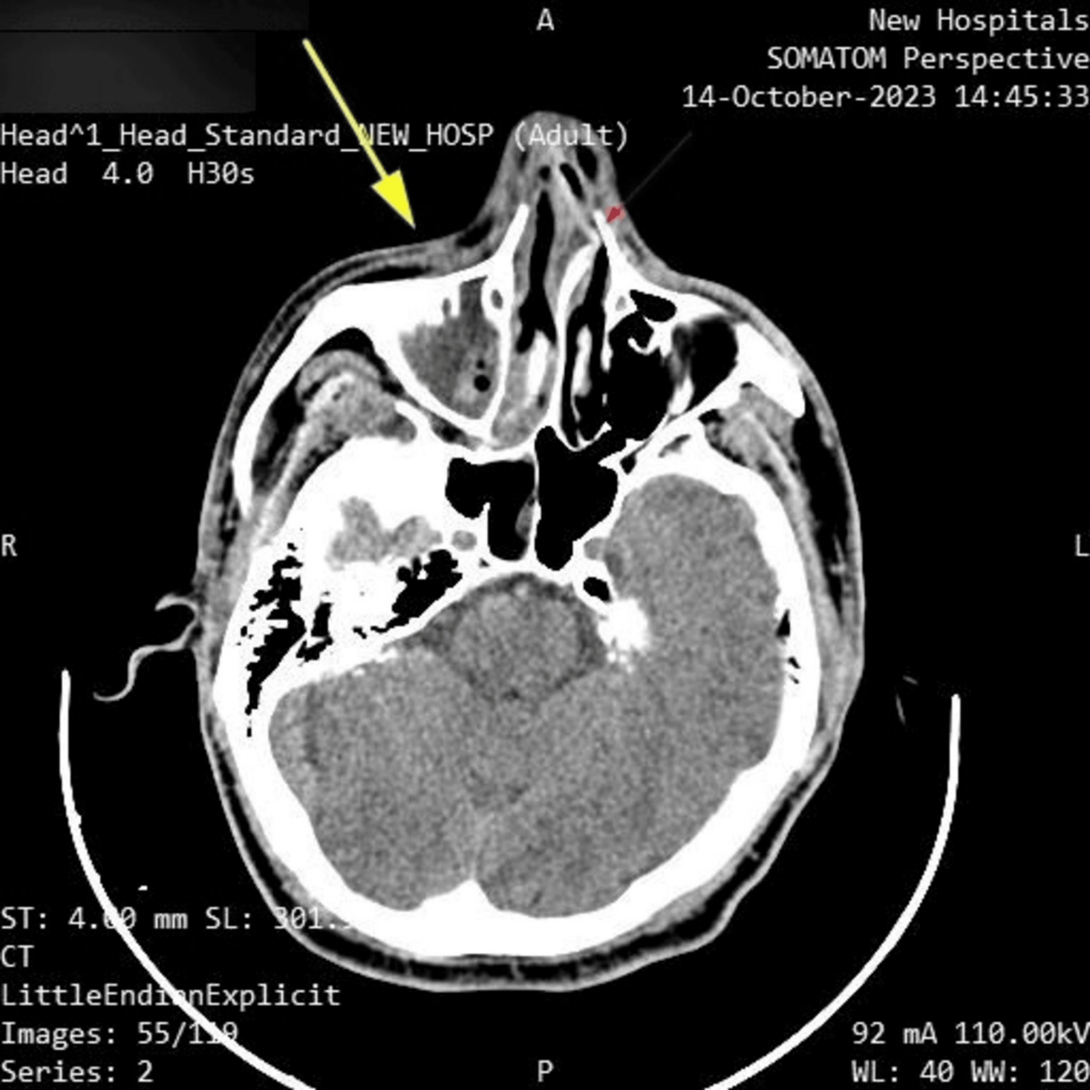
CT head axial Right-sided maxillary sinusitis (yellow arrow) and deformation of the nasal septum (red arrow).
